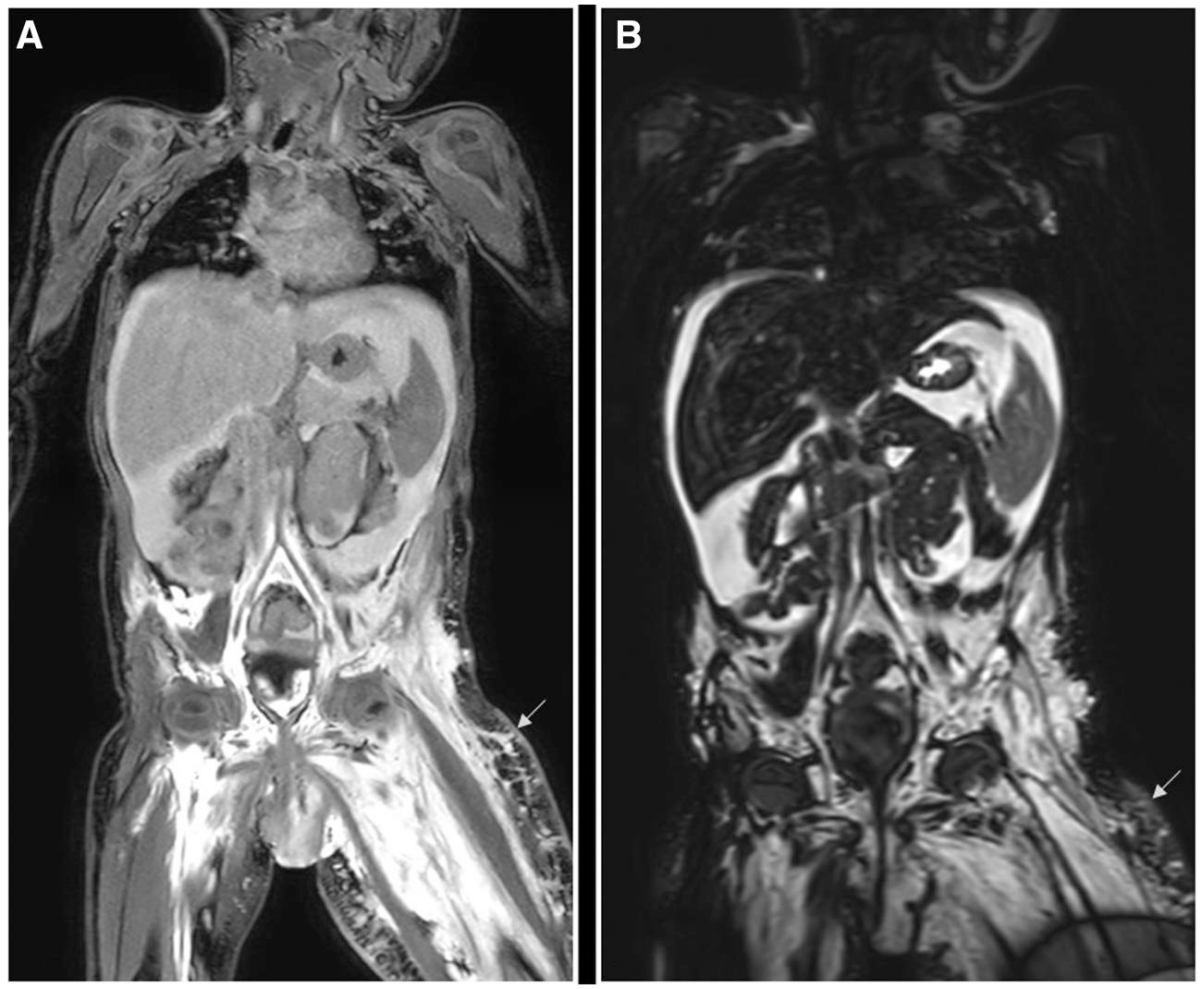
Magnetic resonance lymphangiogram performed at 1.5 T Philips Ingenia, (A) shows 3D-T1-weighted DIXON image acquired after 20 min post-injection of 10 mL gadobenate dimeglumine intracutaneous in bilateral lower limbs and (B) showing coronal VISTA (3D volume isotropic TSE sequence) acquisition in the same patient with a bilateral lower limb lymphovascular malformation, showing extensive dermal backflow, more on the left side (arrows). There is significant oedema in bilateral lower limbs and the vulva region with associated fat stranding and fibrotic changes suggestive of chronic lymphatic obstruction. VISTA: Volume ISotropic Turbo spin echo Acquisition.
